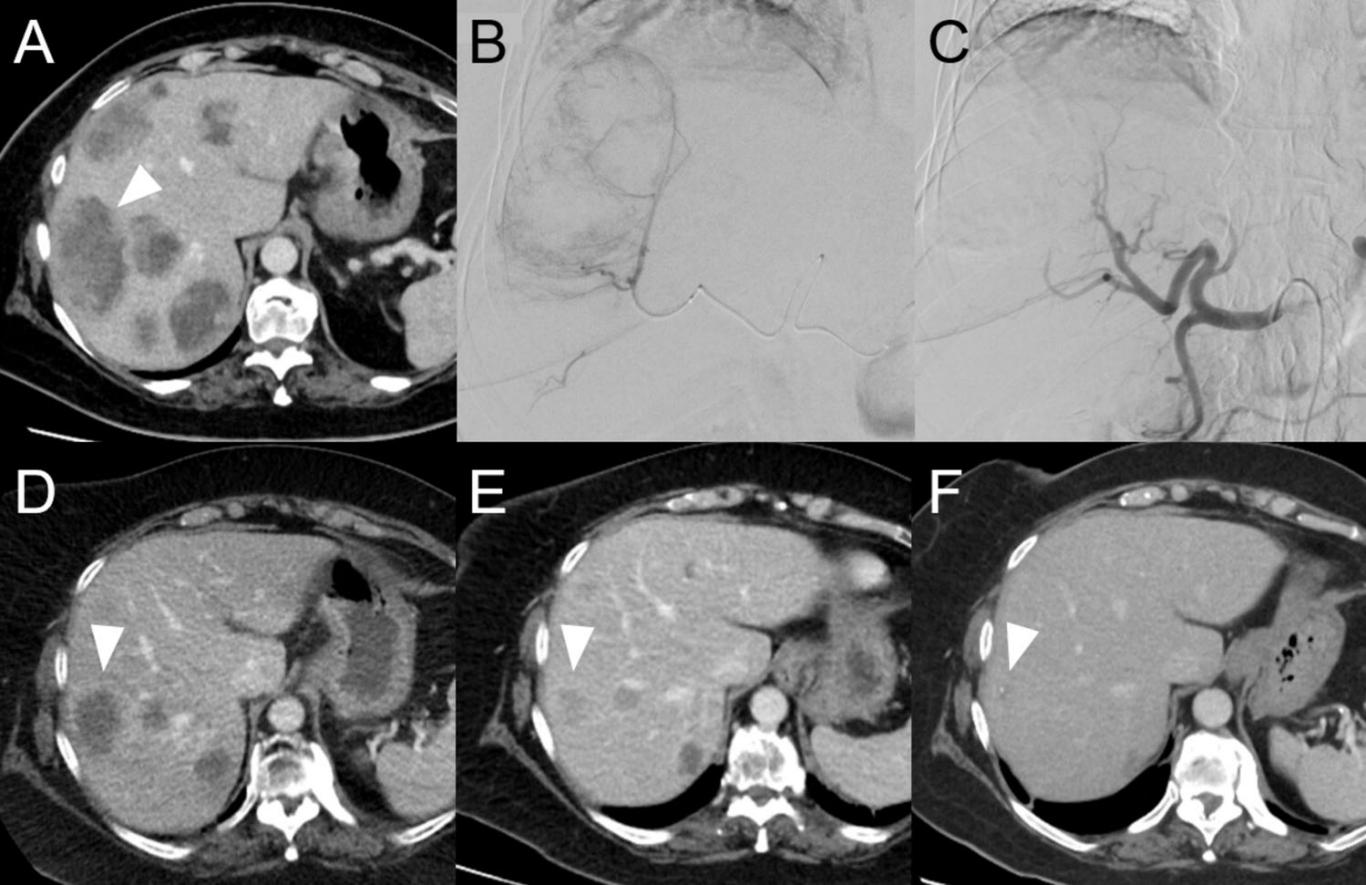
63-year-old female patient (No. 1) experiencing hepatic disease progression under anti-PD1 therapy. A Baseline axial contrast-enhanced computed tomography showing multiple bilobar liver metastases up to 8 cm in diameter (the largest lesion is marked with a white arrowhead). B First TACE procedure performed with the microcatheter (black arrowhead) positioned in the right hepatic artery. C Digital subtraction angiography performed with the guiding catheter in the celiac trunc right after the TACE procedure with no visible tumor blush. D–F Follow-up imaging at 3- D, 6- E, and 18 months after the first TACE F
